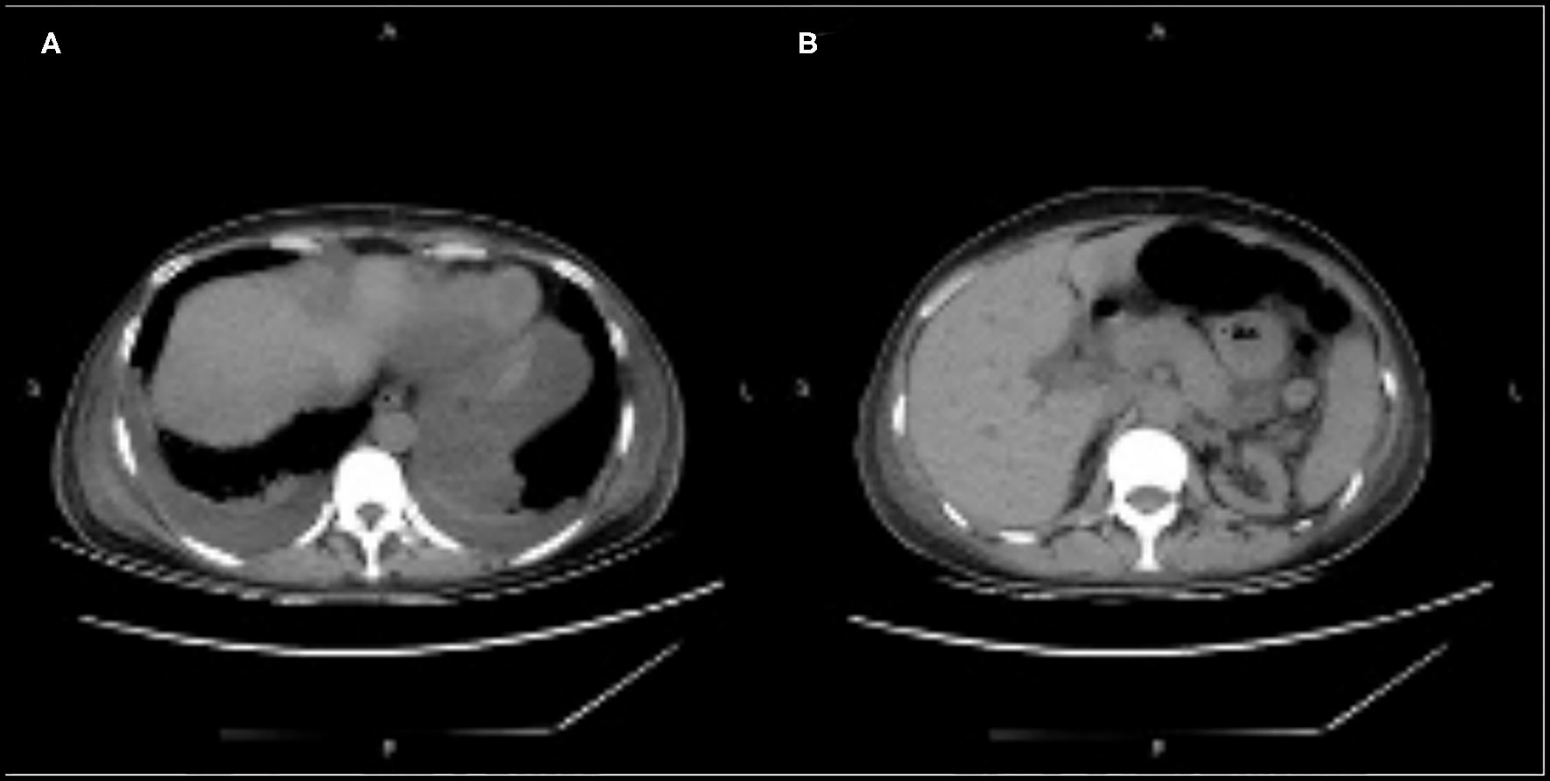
(A,B) Computed tomography showing decreased bilateral pleural effusion and peripancreatic effusion.
